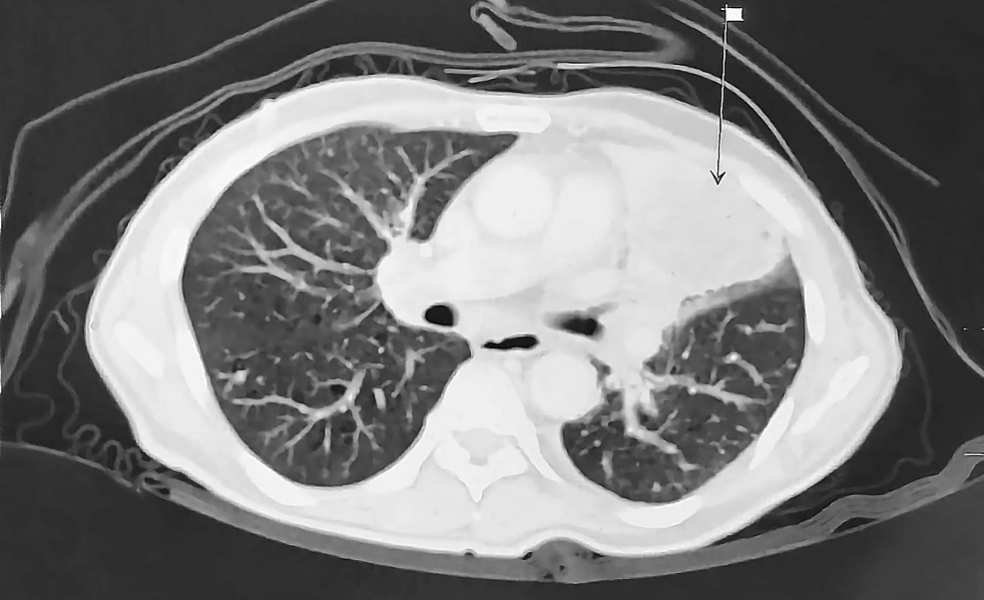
HRCT scan of Patient #1 (2.9× magnification). HRCT: high-resolution computerized tomography
